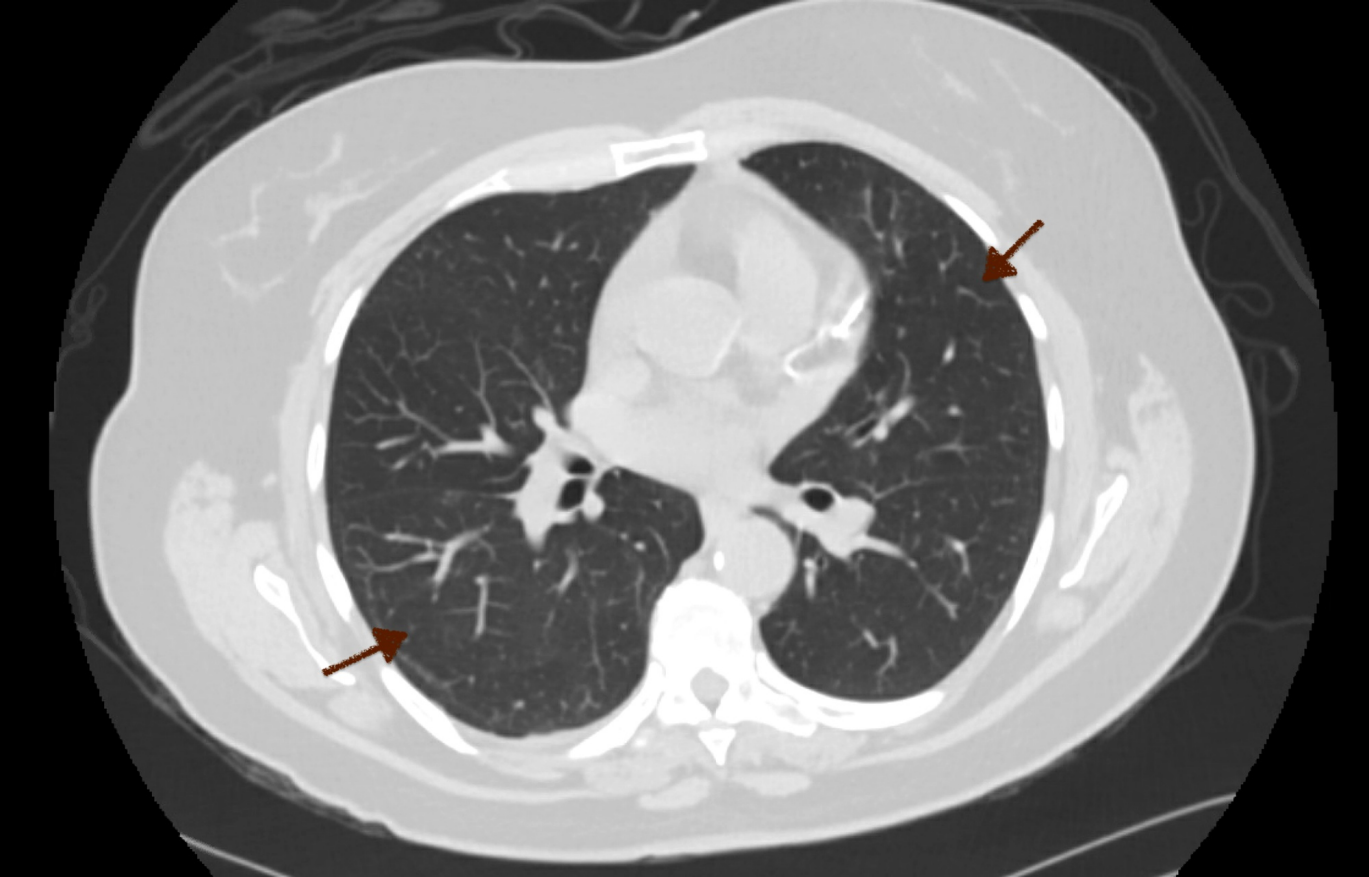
CT Scan No pulmonary opacities noted bilaterally (red arrows). No cardiomegaly appreciated.
